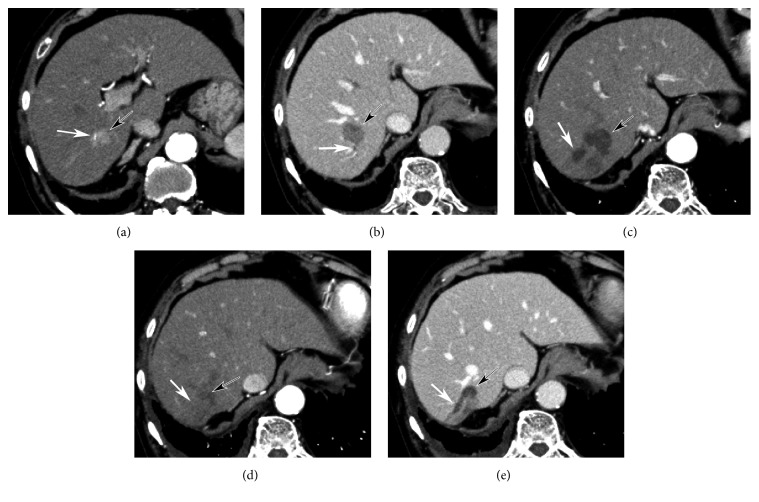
Biloma after RAIB-TACE in an 86-year-old patient with a HCC nodule adjacent to GS. (a) Arterial phase CT showing a hypervascular nodule (black arrow) attached to the superior posterior subsegmental artery (white arrow). (b) Portal phase CT at the different level showing slight biliary dilatation (white arrow) distal from the nodule (black arrow). (c) CT obtained 3 months after RAIB-TACE showing CR of the nodule (black arrow) and biloma (white arrow). No treatment was required for the biloma, as the patient remained asymptomatic. (d) and (e) CT obtained 10 months after RAIB-TACE revealing shrinkage of the biloma (white arrow) and no recurrence of the nodule (black arrow).
